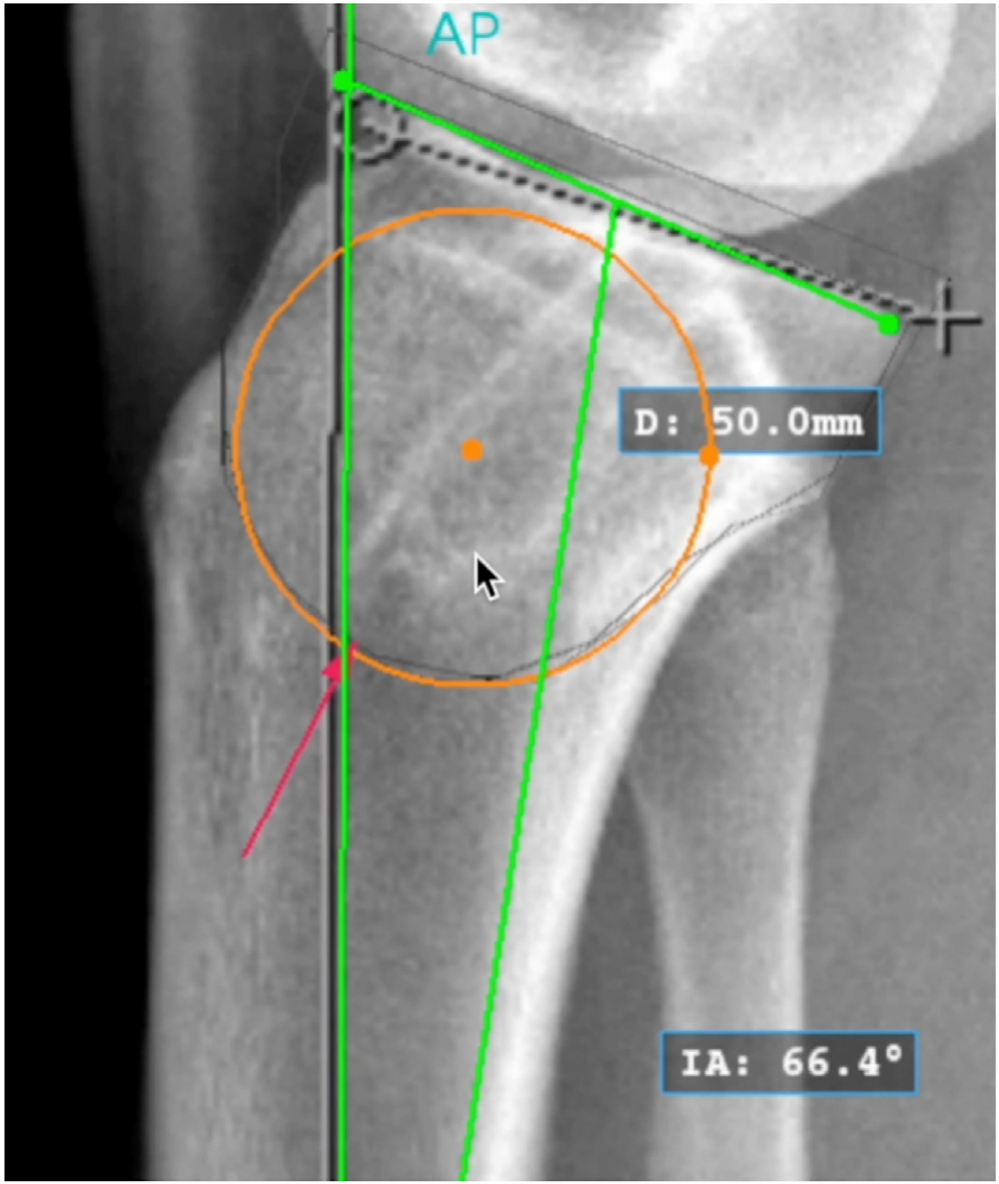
A lateral radiograph of the right knee is used to identify the center of the proximal tibia (orange dot) using a best-fit circle (orange circle). The sagittal slope is measured using the anatomic axis (vertical green line with red arrow) and medial tibial plateau (green line) (IA: 66.4°). (D: 50.0 mm) is the diameter of the best-fit circle. (D, diameter; IA, posterior tibial slope measure.)
